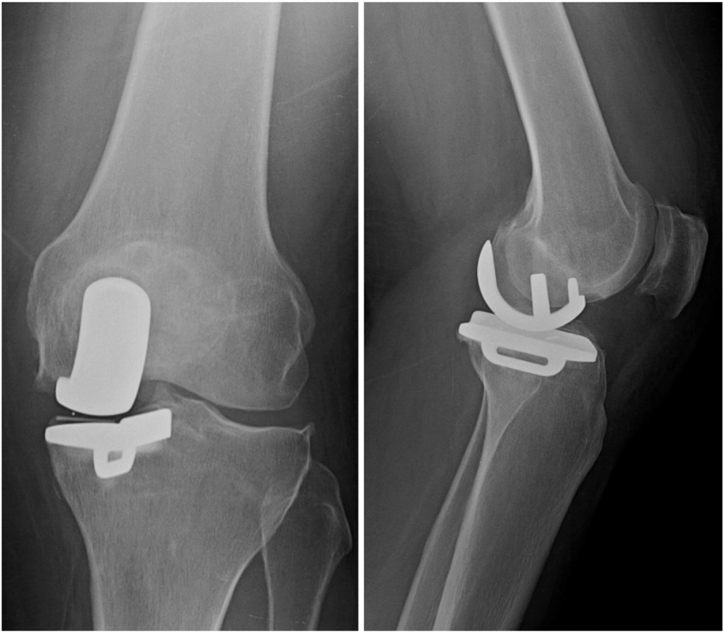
Anterior-posterior and lateral X-rays of the left knee at her 3-month postoperative appointment.
